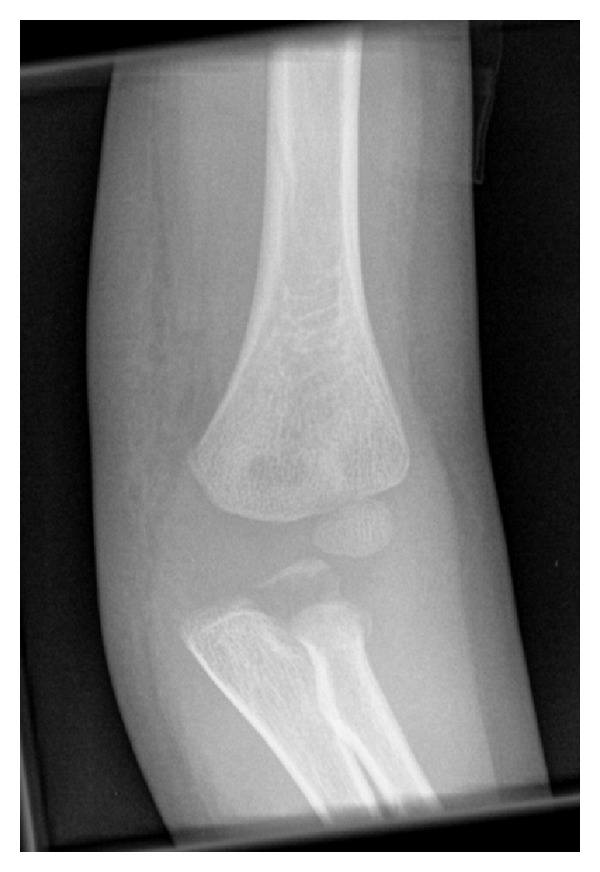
Anterioposterior radiograph of the same elbow twelve days after injury. Bony fragment still visible.
